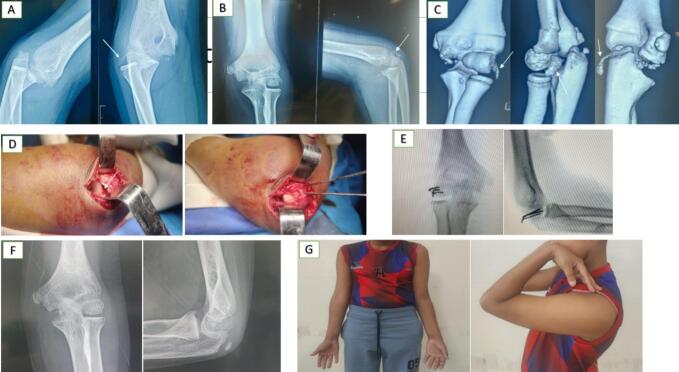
Case 1: Posterior Capitellar Shear Fracture (Type I - Hahn-Steinthal) with Proximal and Lateral Displacement Associated with Elbow Dislocation. (A) Anteroposterior and lateral radiographs at admission reveal elbow dislocation and a posterior fracture fragment (white arrow). (B) Post-reduction anteroposterior and lateral radiographs demonstrate successful reduction, with suspicion of a posterior capitellar shear fracture (white arrow). (C) 3D CT scan confirms the Type I (Hahn-Steinthal) capitellar shear fracture with proximal and lateral displacement (white arrows). (D) Intraoperative images depict the capitellar shear fracture and fixation using K-wires. (E) Postoperative anteroposterior and lateral radiographs show successful reduction and appropriate K-wire placement. (F) Follow-up radiographs at 2 months post-surgery confirm fracture union and anatomical alignment. (G) Clinical photographs at 2 months post-surgery demonstrate full range of motion (ROM) and functional recovery.
